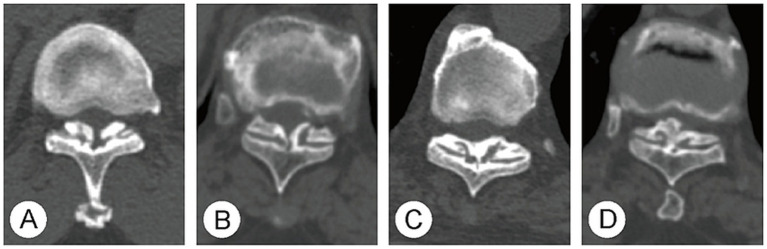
Thoracic OLF was classified into non-fused types (A,B) and fused types (C,D) based on the results of axial CT scans.
